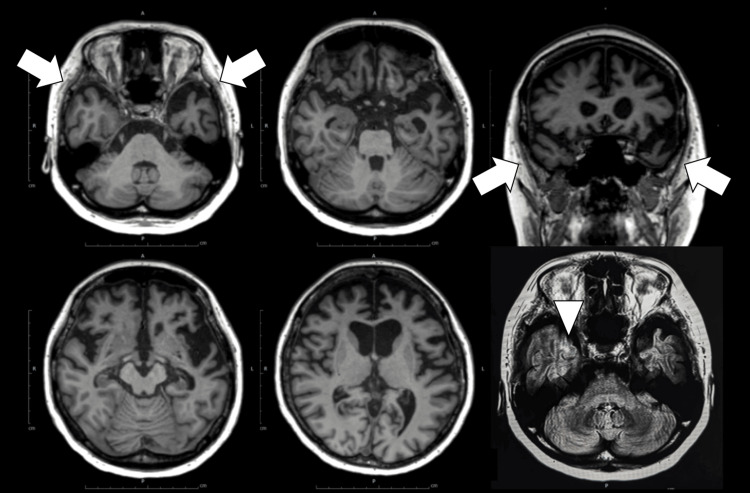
Magnetic resonance imaging findings. Head MRI showing left-dominant atrophy in the frontotemporal and limbic lobes, and insula. Characteristic knife-blade atrophy is visible, along with mild subcortical FLAIR hyperintensity in the temporal pole (arrowhead). Severe bilateral atrophy of the temporalis muscles is also noted (arrows). FLAIR: fluid-attenuated inversion recovery.
